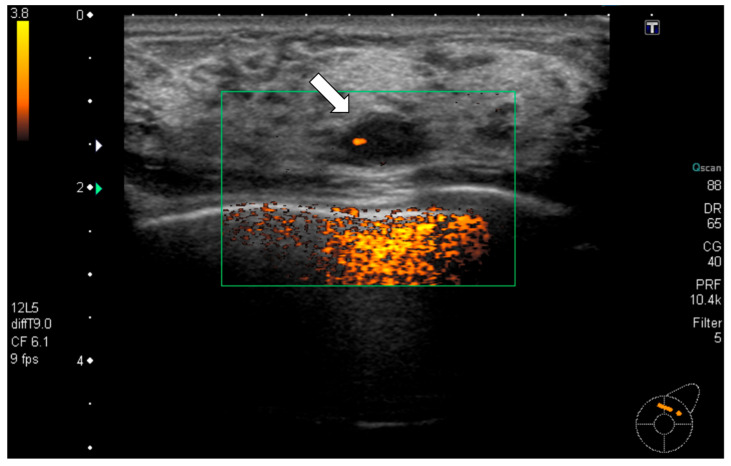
Ultrasonography (initial): A well-defined oval hypoechoic mass measuring 11 mm (arrow) was observed in the upper outer quadrant of the left breast with partial internal vascularity. Ultrasound-guided core needle biopsy was performed for this mass.
